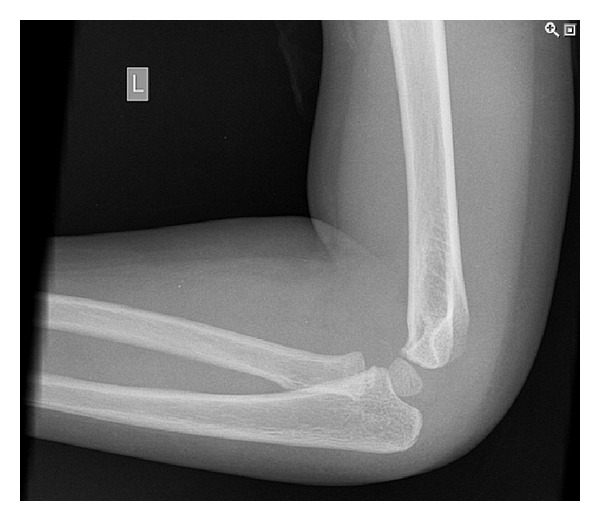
Lateral radiograph of three-year-old boy showing olecranon fracture.
